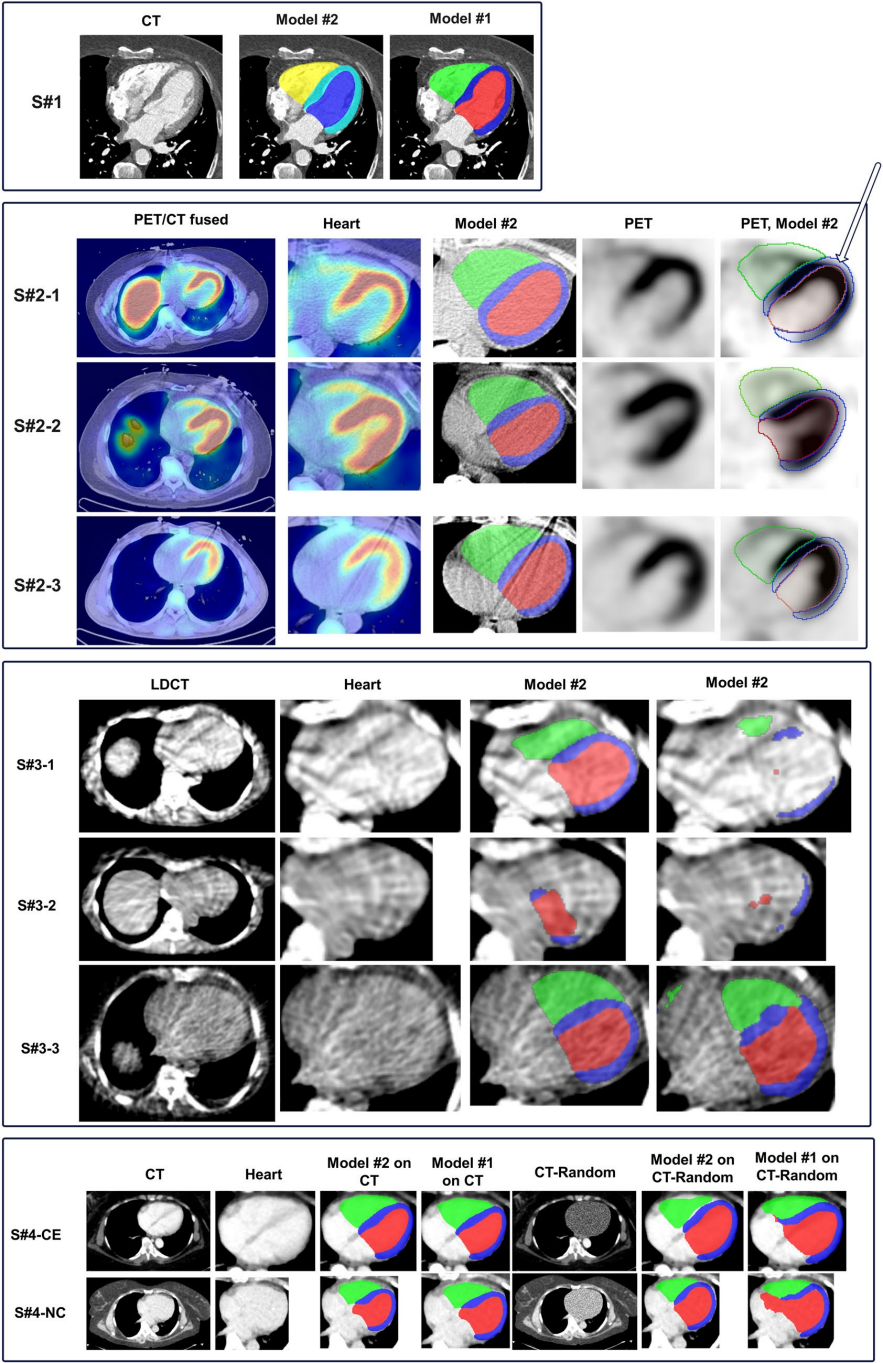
DL CT inferences. From top to bottom sample #1 (S#1) to sample #4 (S#4). S#4–1 windowing (− 110 to 170). S#4–2 windowing (− 60 to 160 HU)
